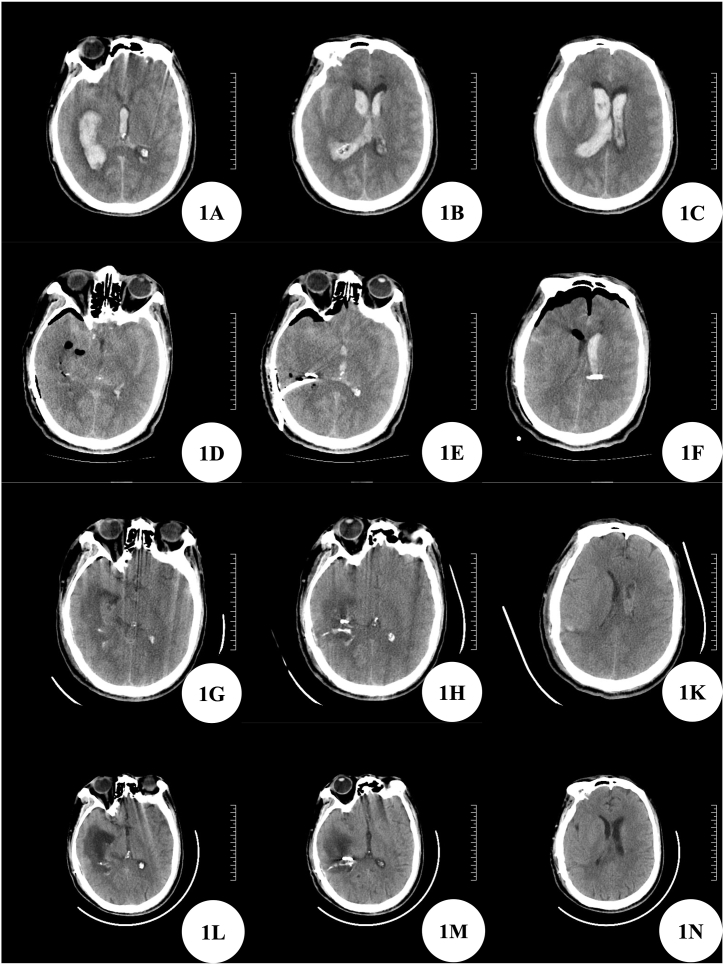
1A–C: Preoperative Brain CT; 1D–F: Brain CT on the day after operation; 1G–K: On the 8th day after operation, Brain CT was performed one day before lumbar cistern drainage; 1L–N: Brain CT before discharge.
